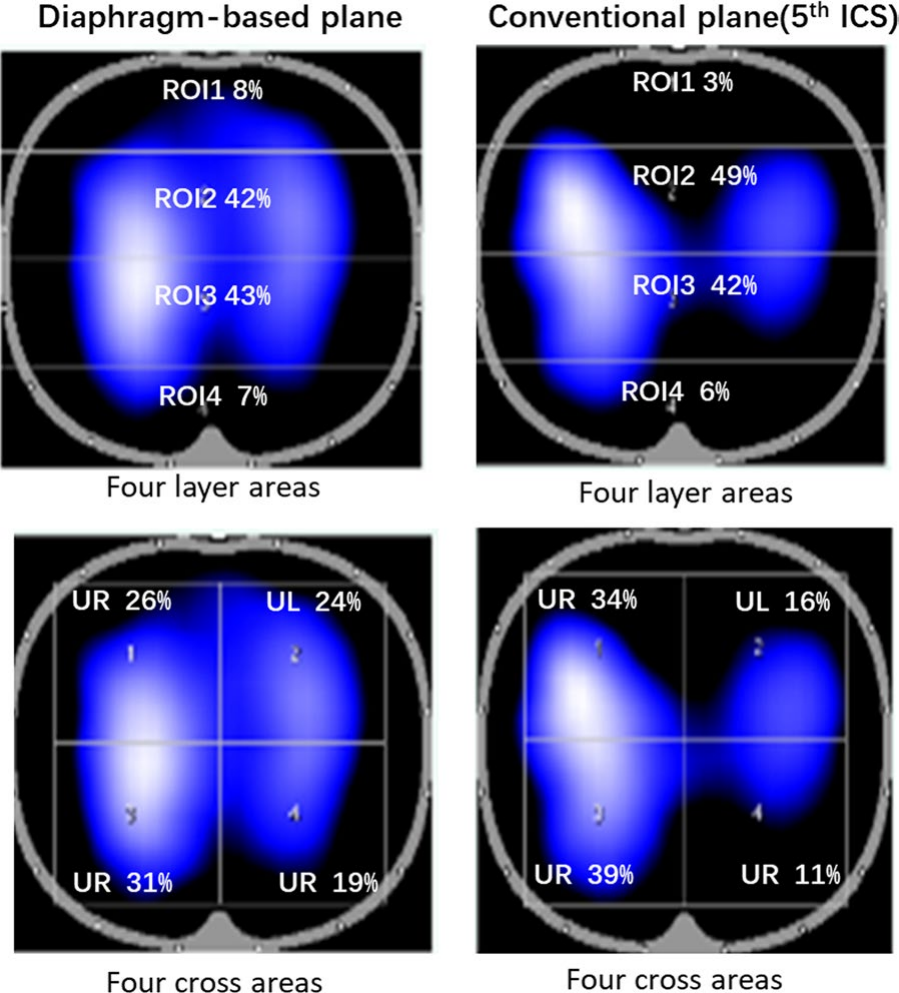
Difference of lung ventilation image in a diaphragm-based EIT-belt plane and fifth intercostal space plane. The diaphragm-based EIT-belt plane by ultrasound had a bigger area of ventilation region (eyeballing method) than 5th ICS plane
